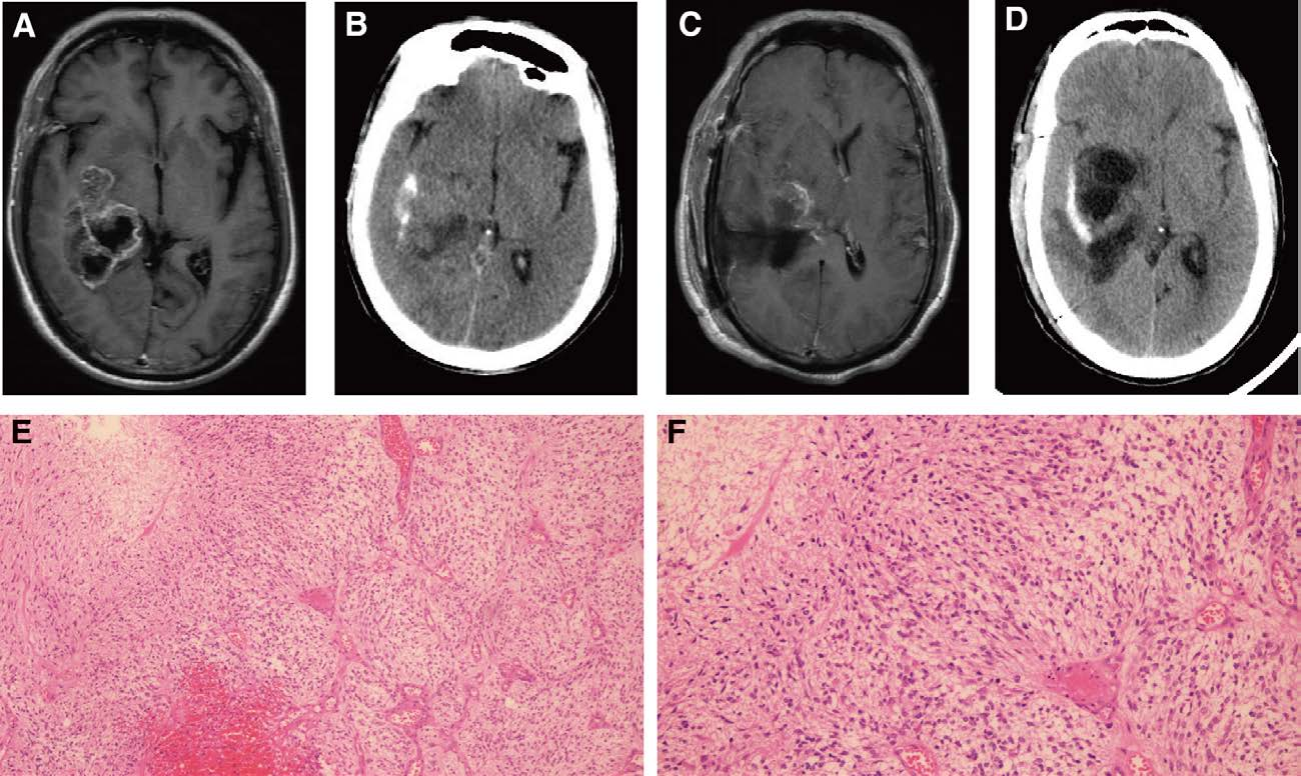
Clinical data of patient number 1. (A) MRI shows patchy hypodense lesions in the right temporal insular lobe, basal ganglia, and corpus callosum, with ring enhancement. (B) CT shows cord-like calcification in the right temporal lobe. (C) Postoperative MRI indicates residual enhancing lesions in the basal ganglia region, with midline structures shifted to the left. (D) Postoperative CT shows residual cord-like calcification in the right temporal lobe. (E, F) Pathology shows spindle-shaped/oval-nuclei tumor cells, with high cellularity and moderate atypia. Pseudopalisading necrosis and glomeruloid microvascular proliferation are observed (left, ×100). Tumor cells exhibit moderate atypia and visible mitotic figures, along with pseudopalisading necrosis and glomeruloid microvascular proliferation (right, ×200). CT = computerized tomography, MRI = magnetic resonance imaging.
